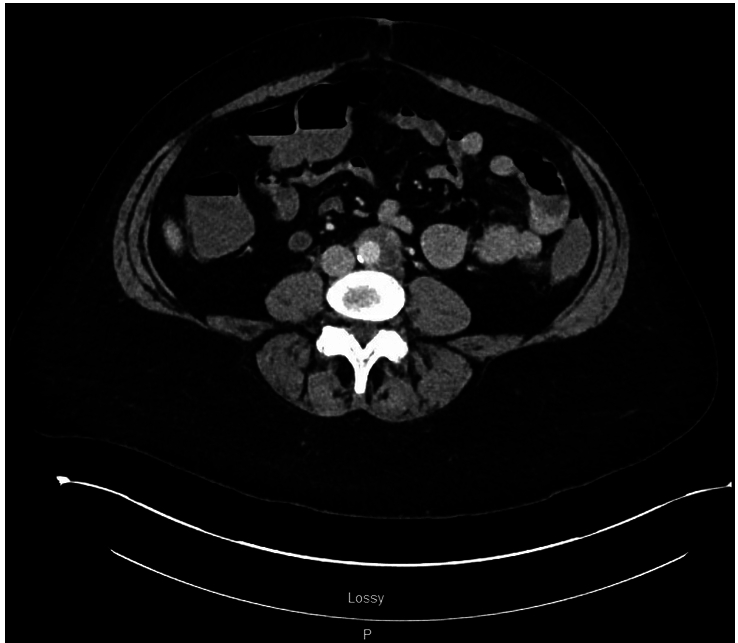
Computed tomography abdomen and pelvis revealing crescentic soft tissue infiltration in the distal abdominal aorta with surrounding inflammatory stranding extending to the left common iliac artery.
